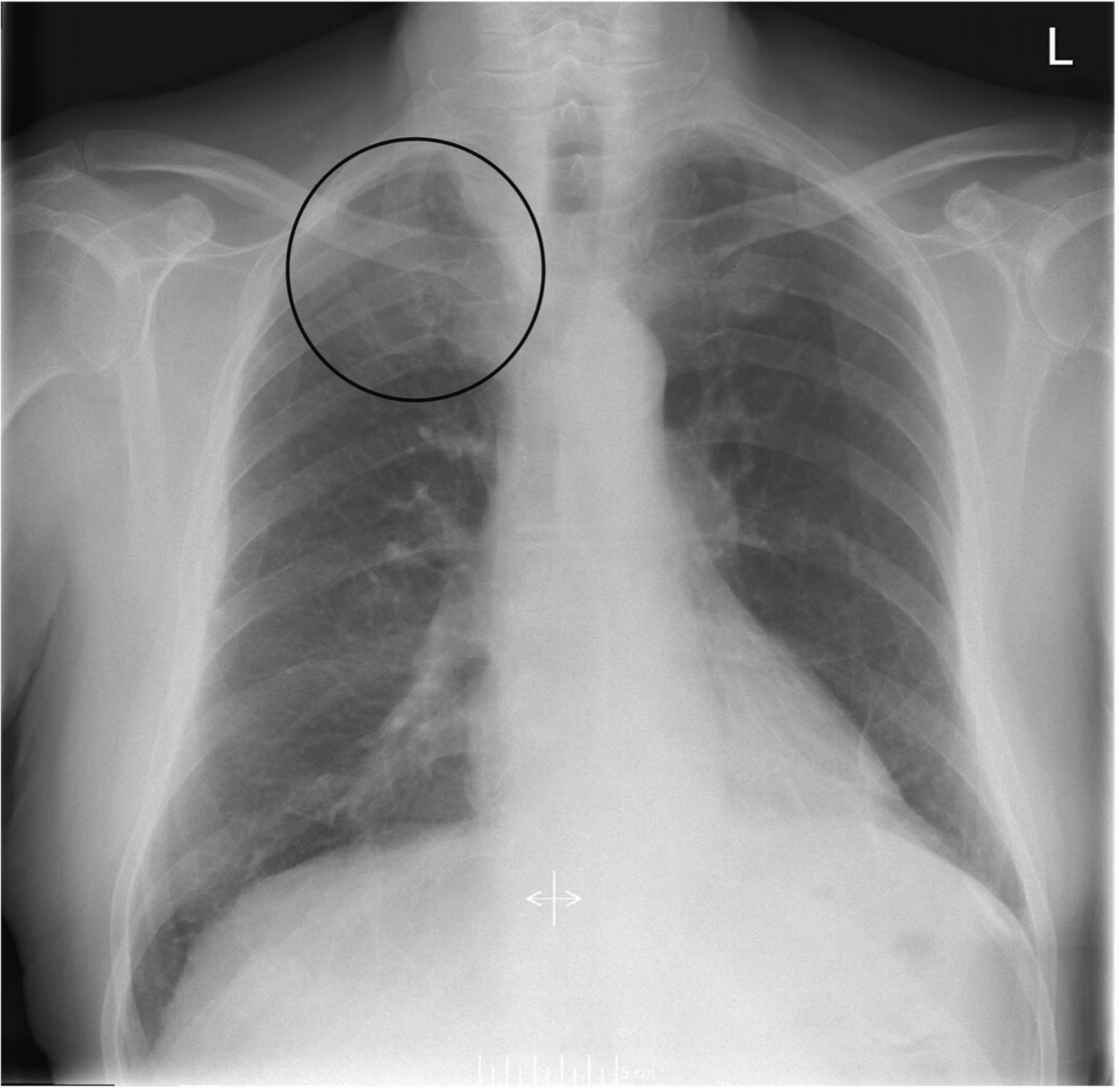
Chest radiograph with subtle opacification in the right upper zone (circle)
